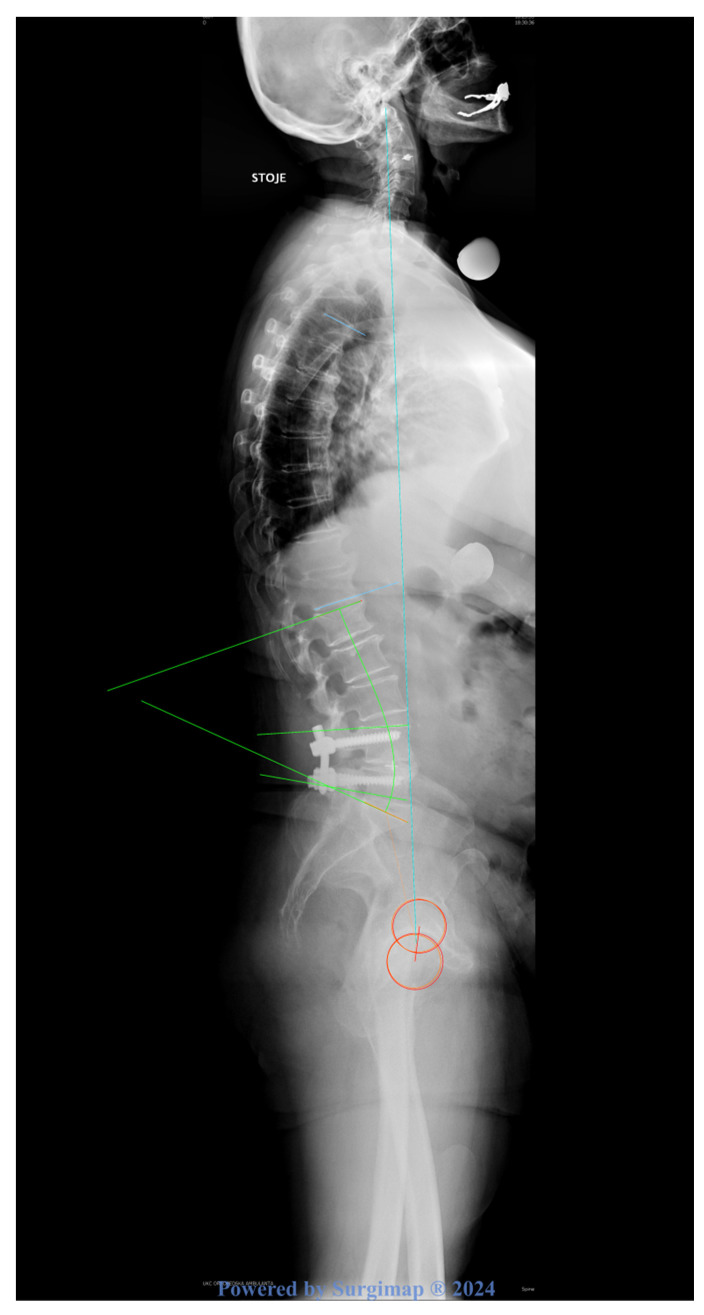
Example of measured parameters defined in Table 1, on full-standing lateral radiograph.
